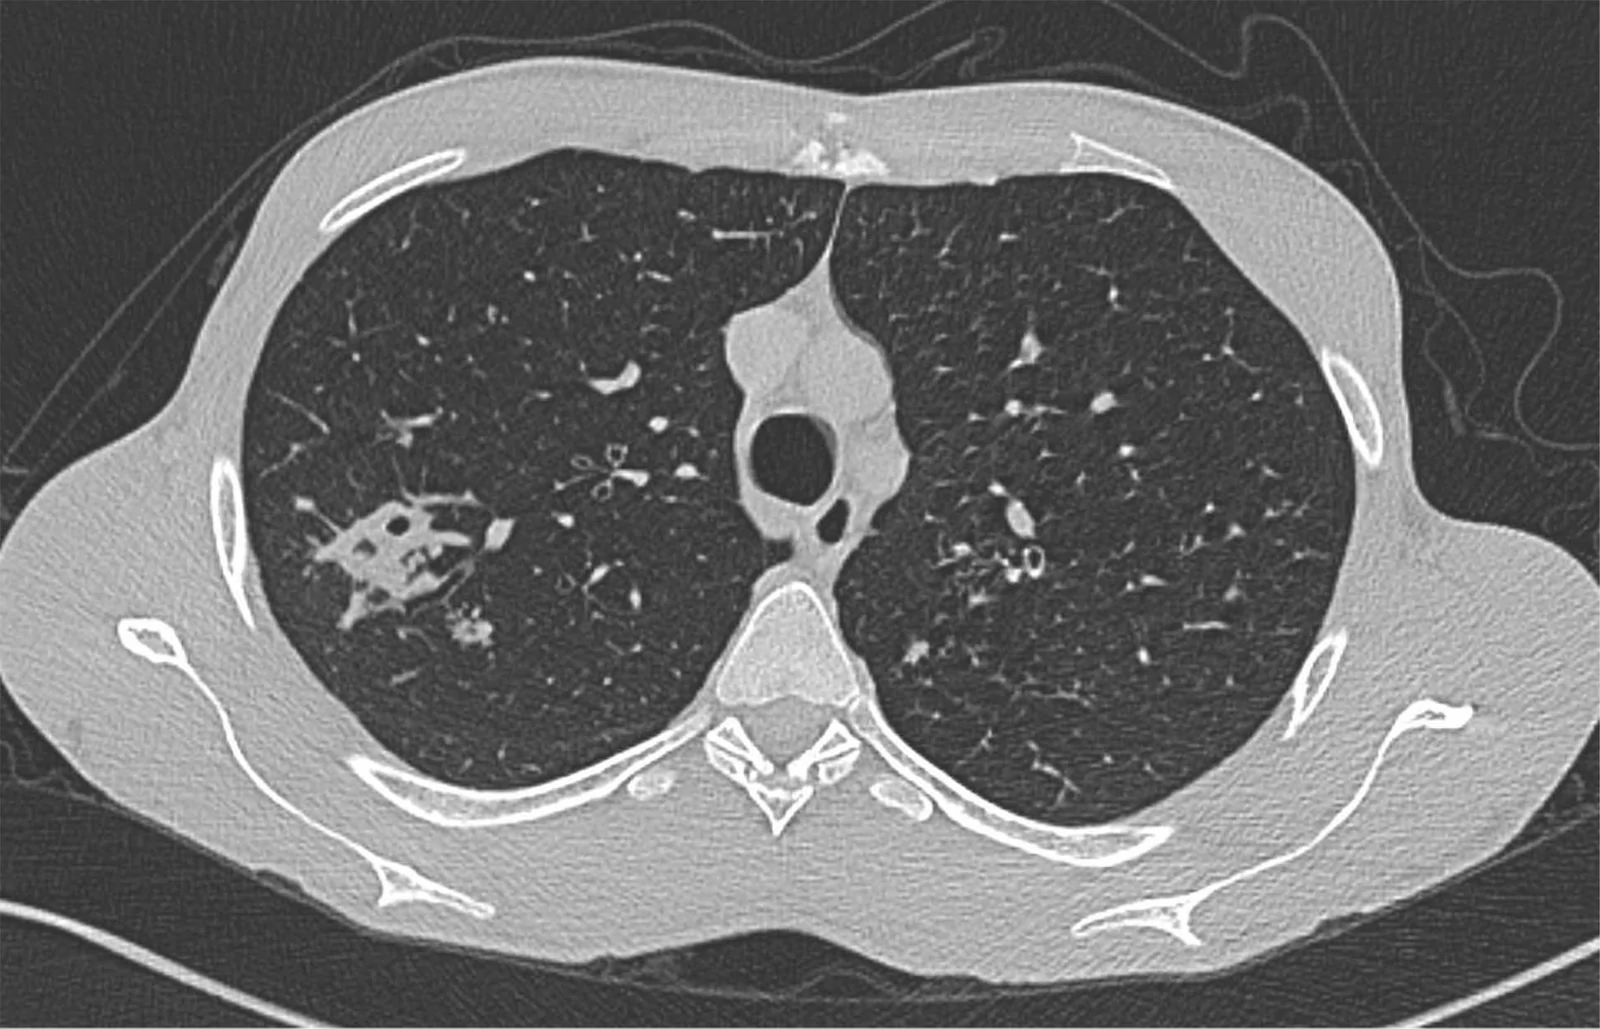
Axial CT scan of the chest showing cavitated nodule in the dorsal segment of the right upper lobe, surrounded by a few micronodules.
